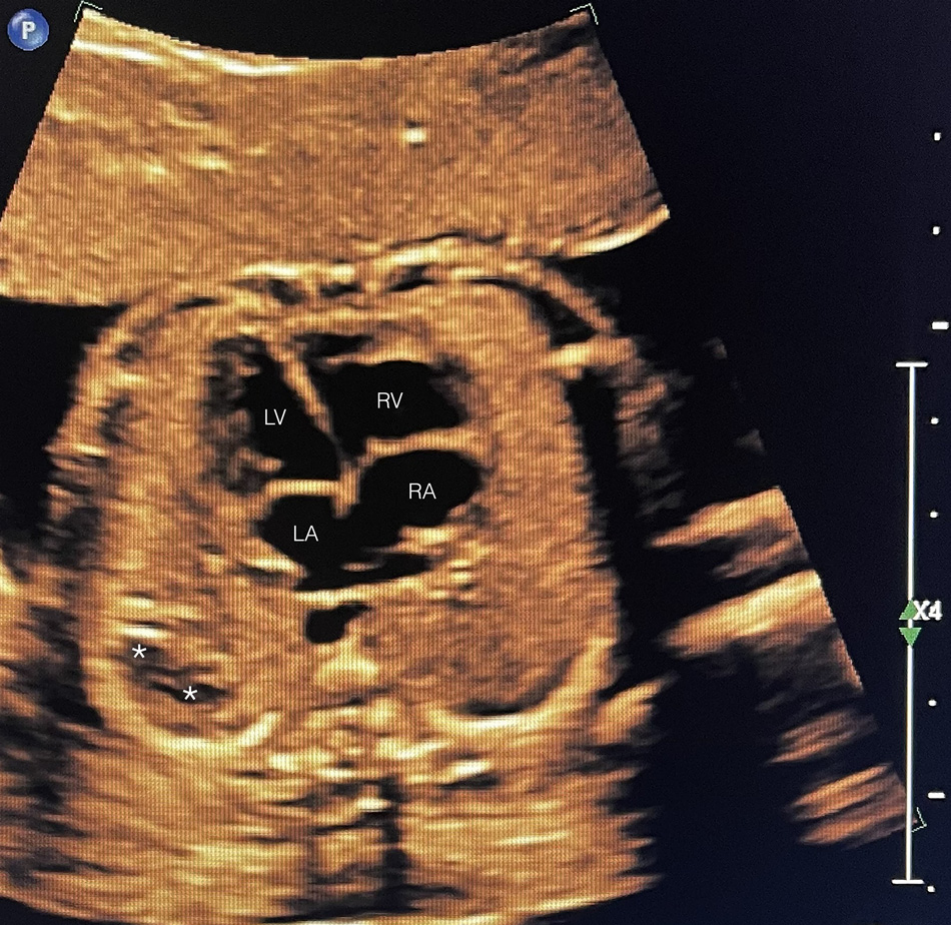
Axial sonographic image of the fetal thorax at the level of the 4-chamber heart at 26 weeks’ gestation. Note the essentially normal-positioned fetal heart representing spontaneous resolution of previously depicted marked displacement to the right, dextroposition. Note also the now normal appearing right fetal lung (previously compressed, which was barely visible at earlier 21 weeks’ gestation (compare with Fig. 2).
